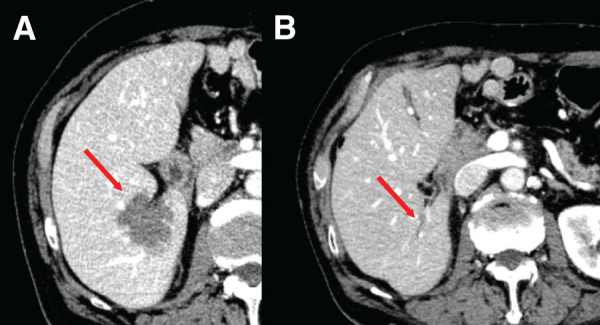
Contrast-enhanced CT images related to RFA. (A) Contrast-enhanced CT image obtained immediately after RFA of the liver metastasis. (B) Contrast-enhanced CT image showing no evidence of local recurrence more than 3 years after RFA of the liver metastasis. RFA, radiofrequency ablation
